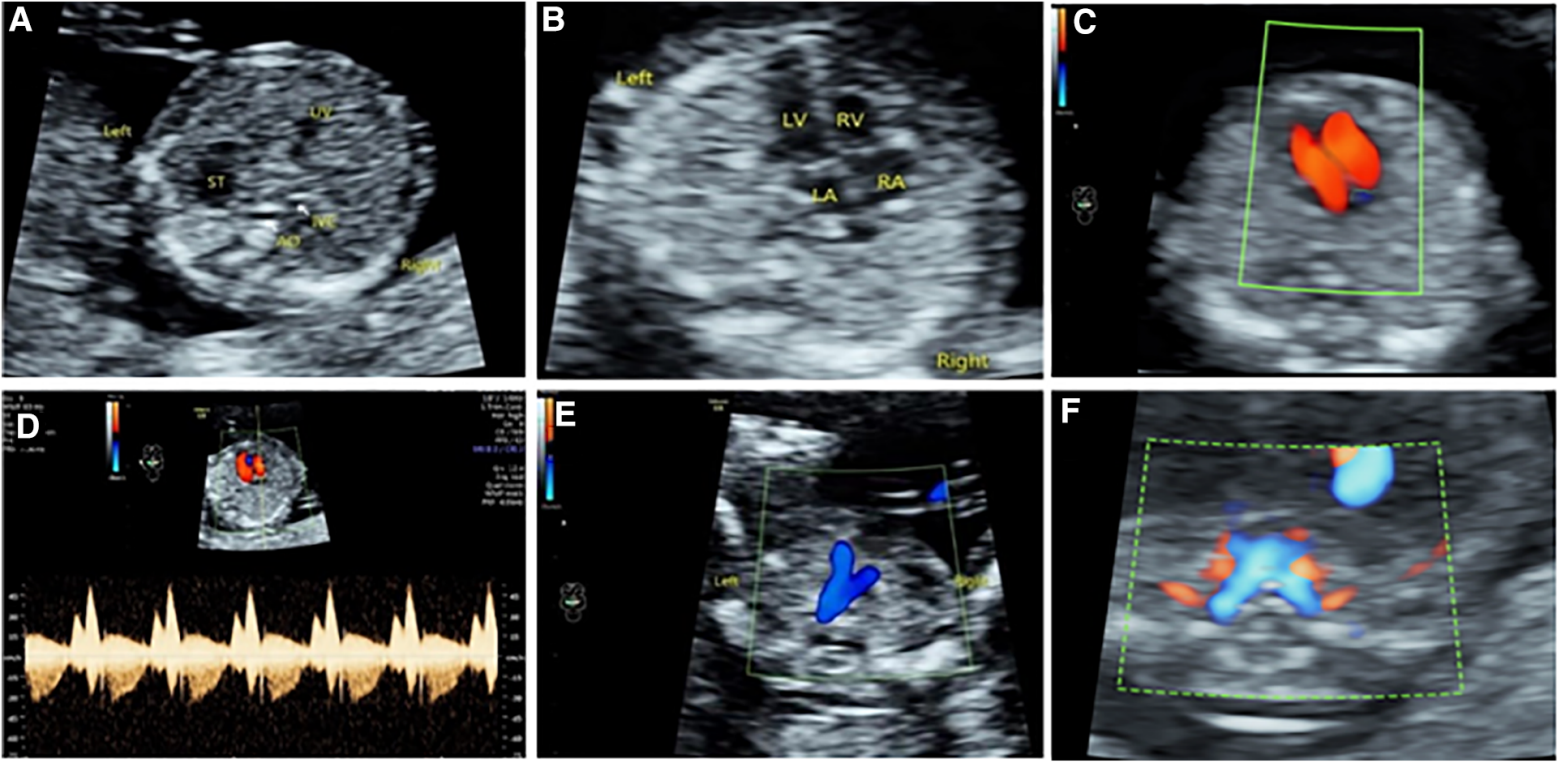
Four-section approach in early pregnancy. (A) Upper abdominal transverse section. (B–D) Two-dimensional, color Doppler, and tricuspid spectrum Doppler ultrasound of 4CV section. (E) 3VT section. (F) Bilateral subclavian artery section.
